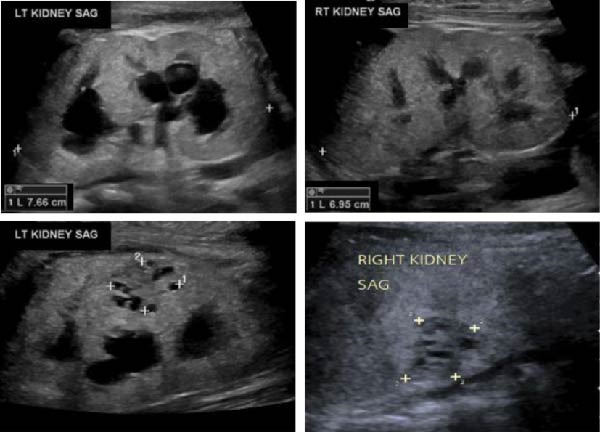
Renal ultrasound showing bilateral enlarged echogenic kidneys with loss of corticomedullary differentiation. Left moderate hydronephrosis. Bilateral cystic, solid lesions in the interpolar region with minimal internal vascularity in Color Doppler Images (not shown; Case 1).
